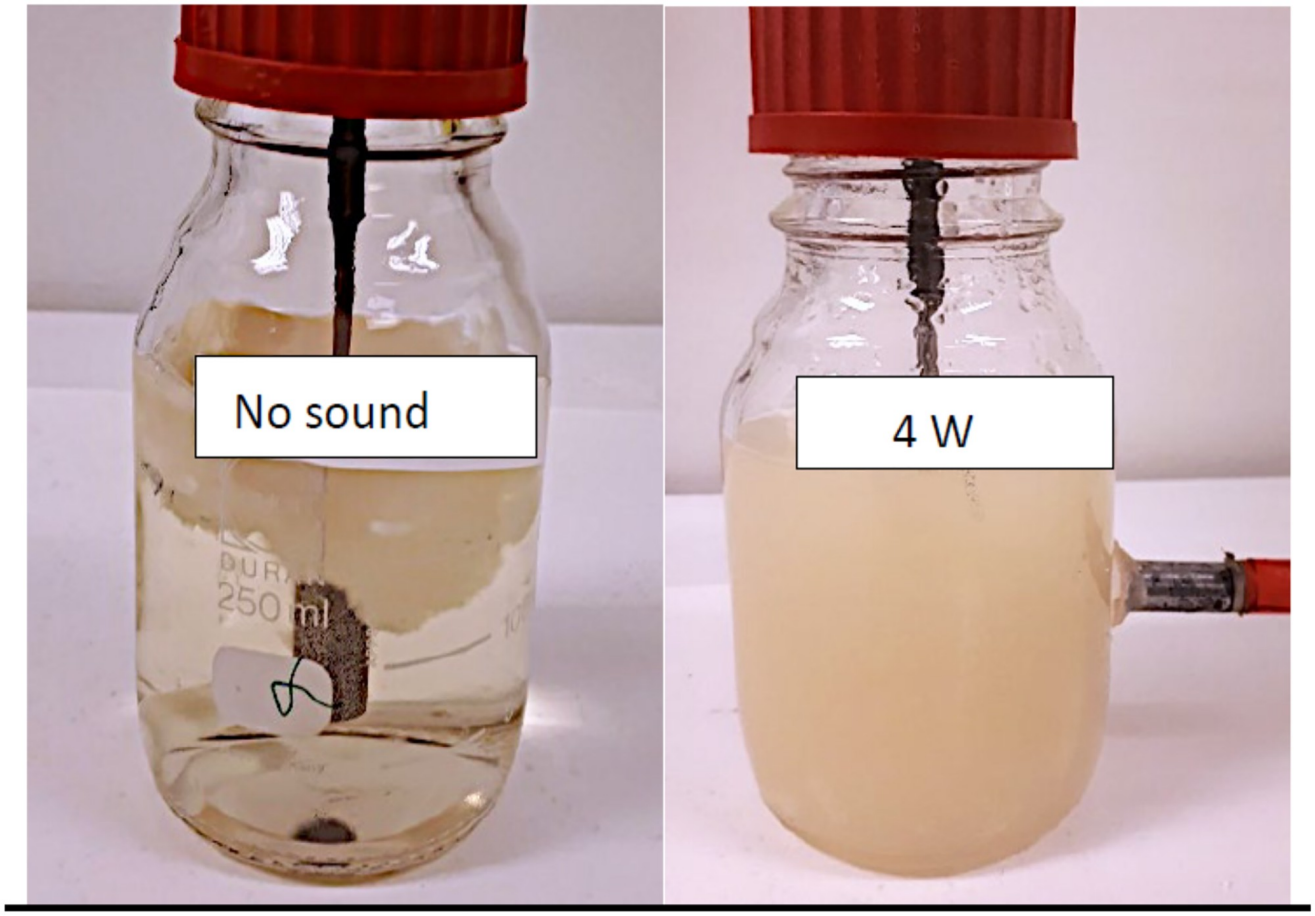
Morphological changes to *F*. *oxysporum* cultures resulting from increased ultrasound power.
